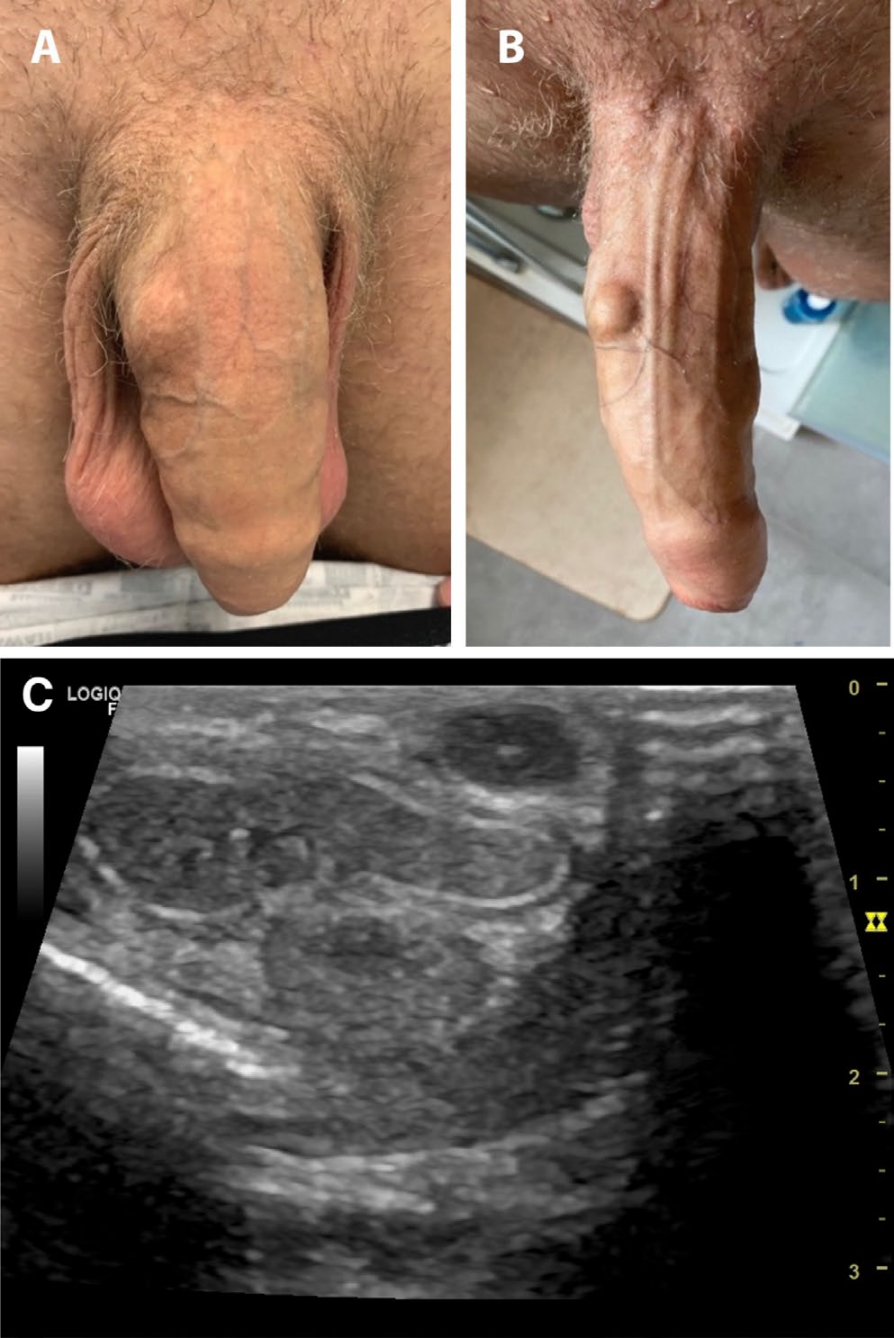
Second case. Nodule on the right lateral part of his penis in: (A) flaccid state, (B) erect state, (C) ultrasound image.
